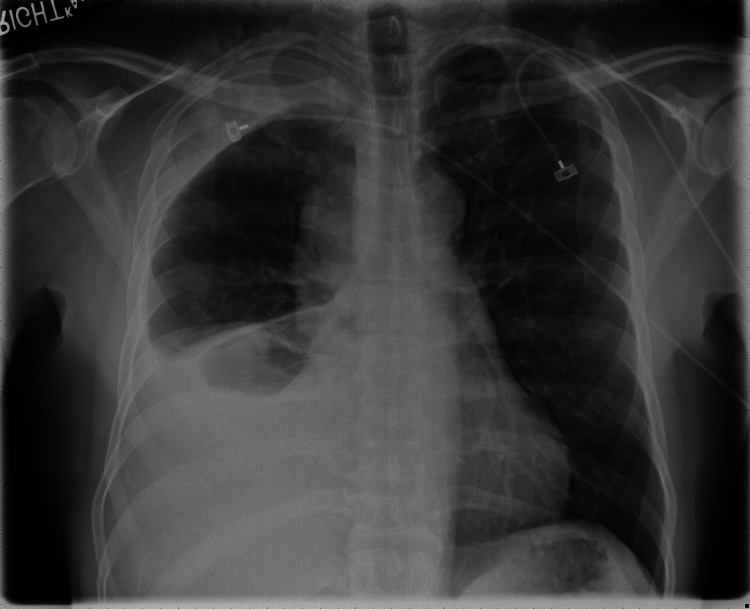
Chest X-ray Chest X-ray showing large unilateral right pleural effusion.
